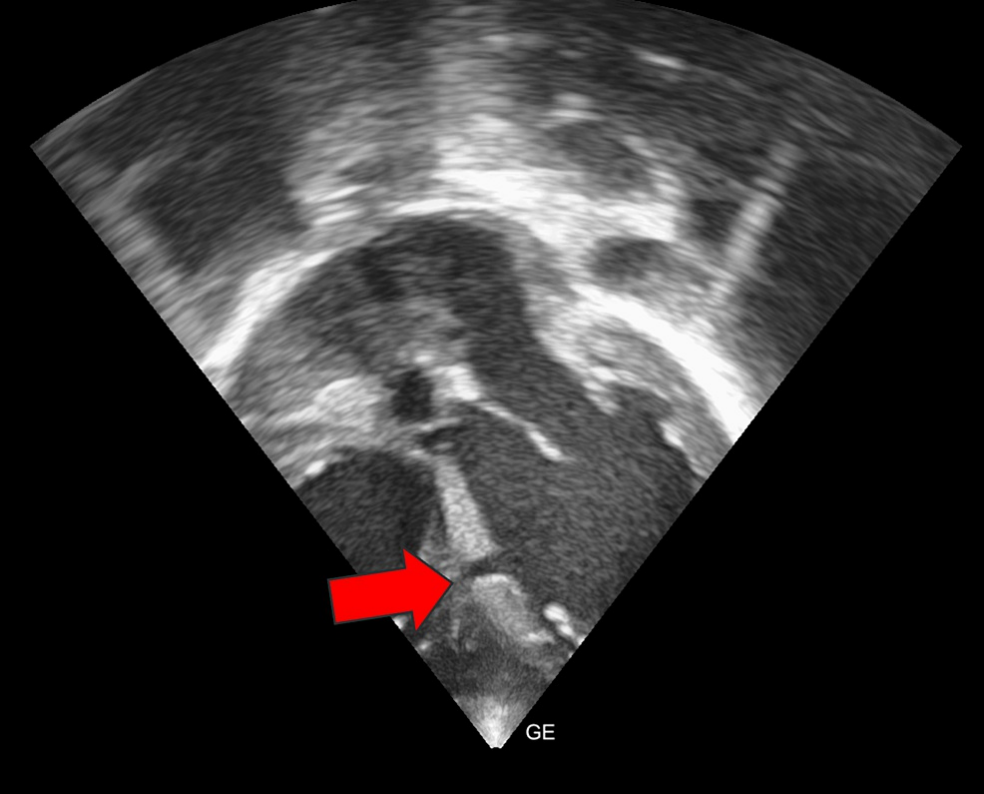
A 2D echocardiogram revealing a ventricular septal defect (red arrow)
